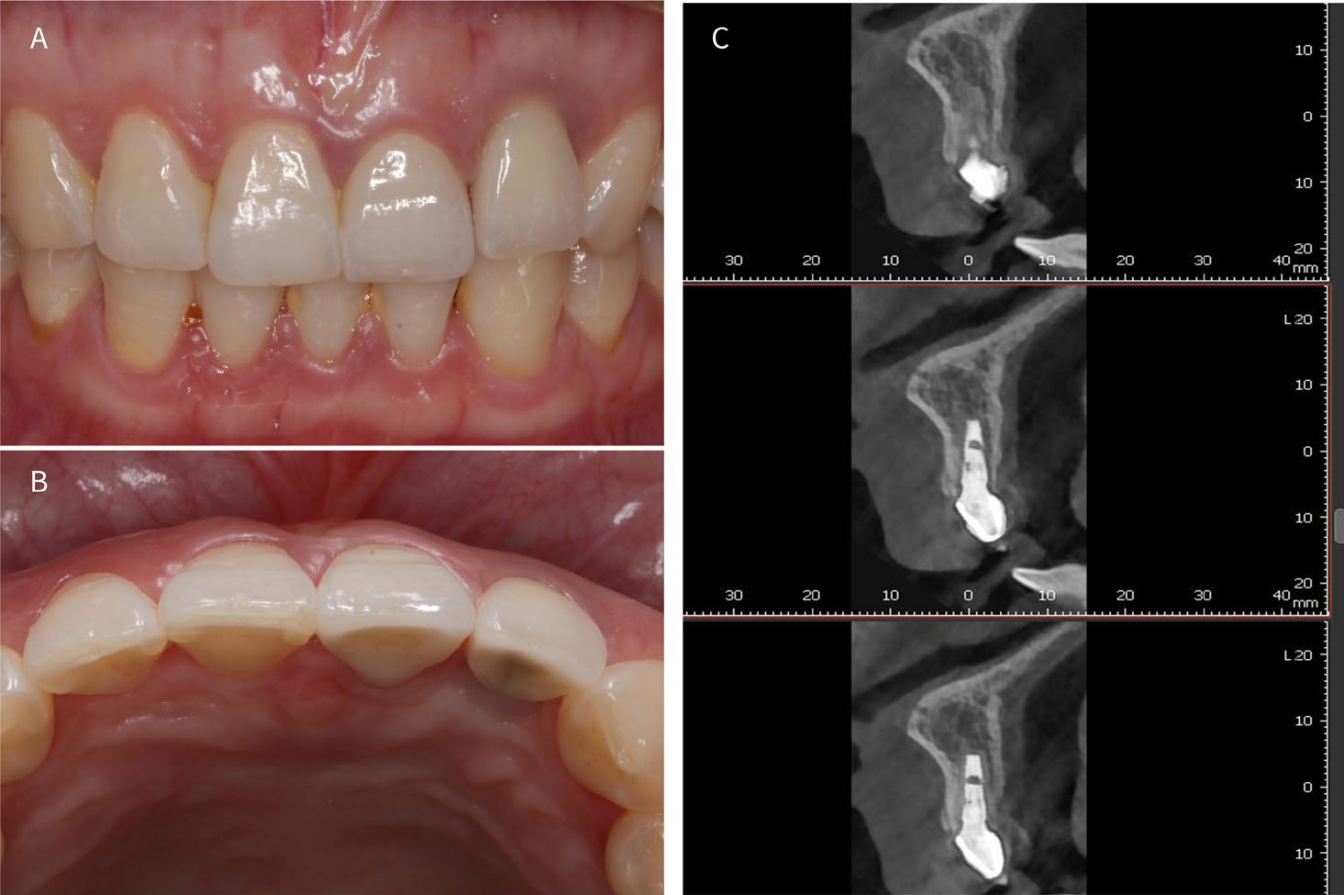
(A, B) Two‐year post‐restoration follow‐up intraoral views; (C) Two‐year follow‐up cone beam computed tomography (CBCT) revealed no significant hard‐tissue resorption observed.
